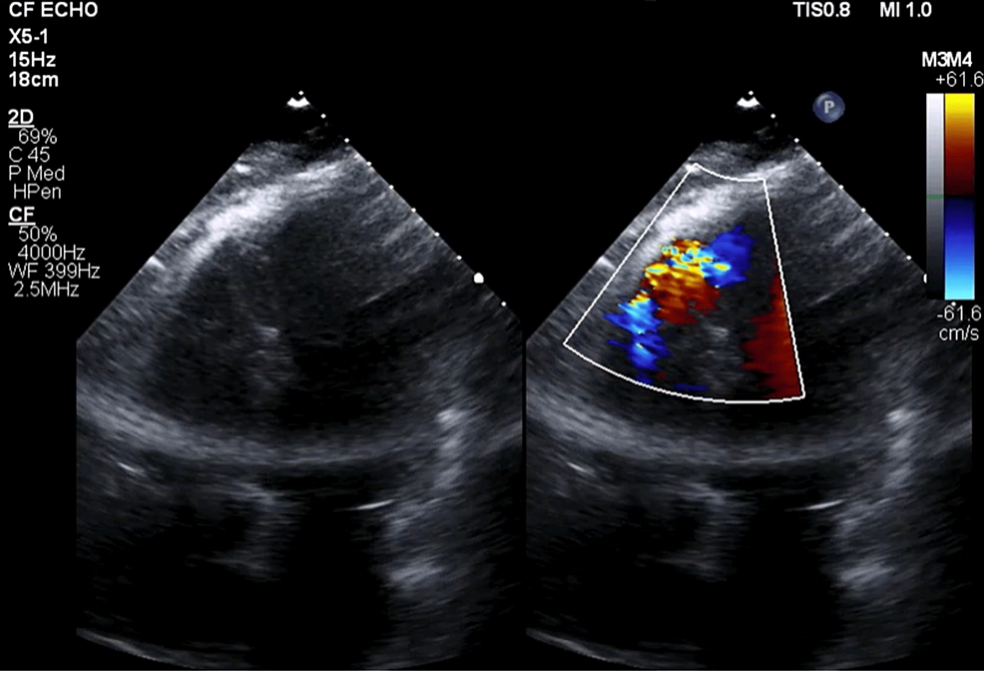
TTE in an apical four-chamber view, showing VSD with left-to-right shunt on Doppler imaging TTE: Transthoracic echocardiogram; VSD: Ventricular septal defect.
